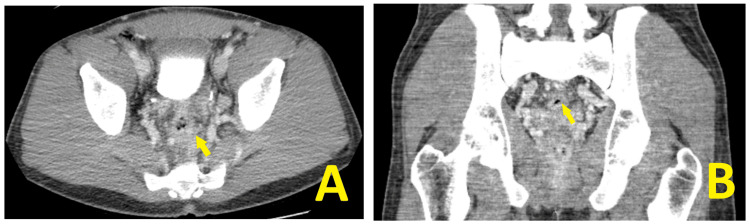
Contrast-enhanced axial and coronal CT of the pelvis A. Contrast-enhanced axial CT of the pelvis showing interval improvement/decrease inflammatory/infectious proctitis (yellow arrow). B. Contrast-enhanced coronal CT of the pelvis shows a similar decrease in proctitis (yellow arrow), without complete resolution of mesenteric and retroperitoneal lymphadenopathy.
